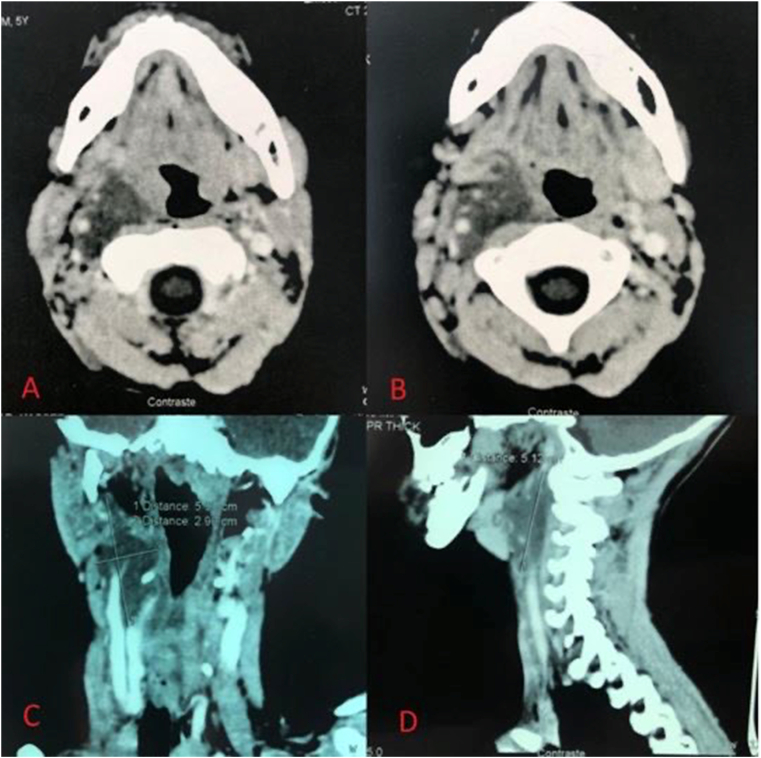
CT-scan showing an irregular hypodense mass, laterally to the -larynx, crossed by the jugulo-carotid vessels. A-B: Axial cut. C-coronal. D-Sagital cut.
